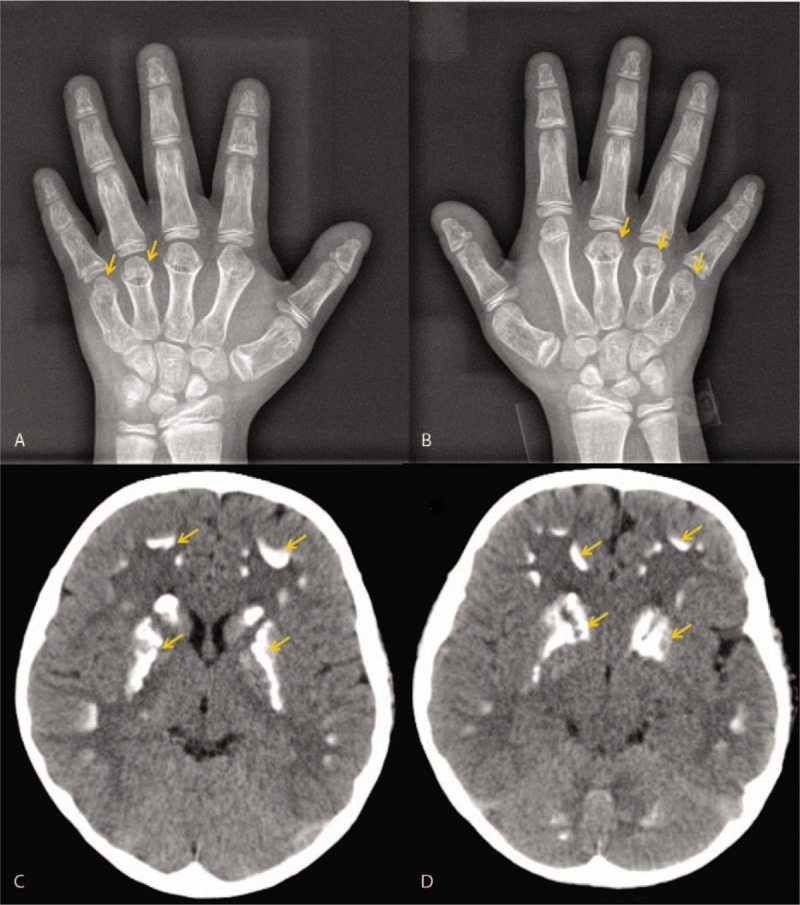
Radiograph of the hands (A: left hand, B: right hand) showing shortened metacarpals (arrow). Cranial computed tomography scan (C, D) demonstrated bilateral calcifications in various regions of cerebrum and cerebellum, especially the basal ganglia (arrow).
